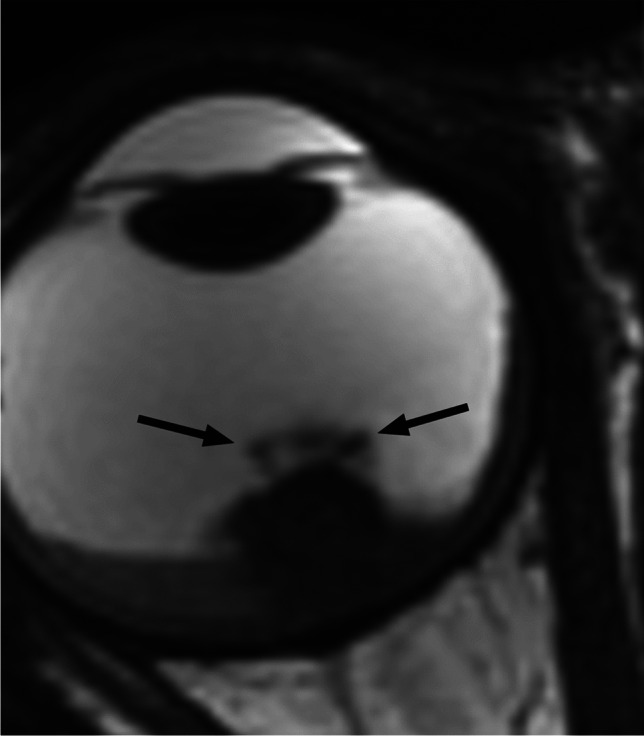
High resolution axial T2-weighted image of the globe shows retinoblastoma with endophytic growth into the vitreous chamber, including vitreous seeding (arrows)
